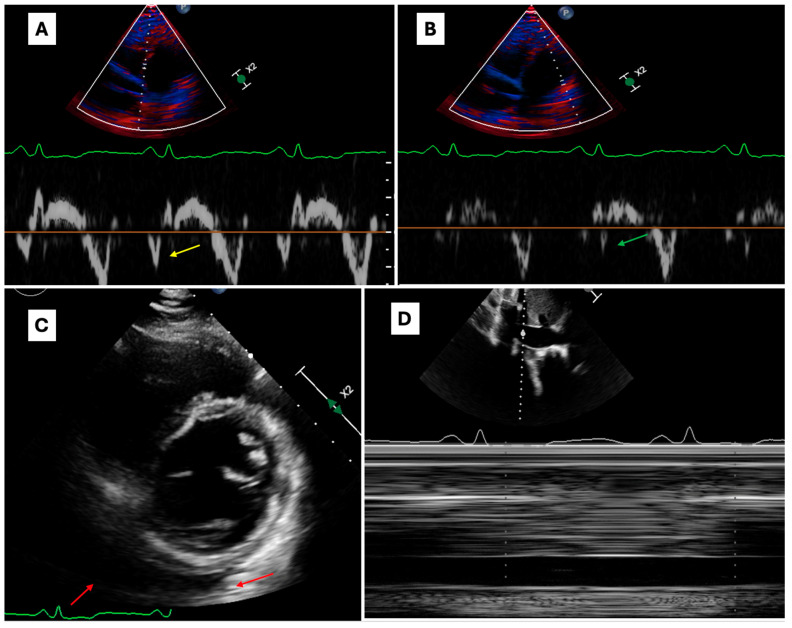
Echocardiographic findings of a patient with constrictive pericarditis. Echocardiographic findings of a patient with long-standing worsening right-sided heart failure. Panels (A,B) show an “annulus reversus” pattern on tissue Doppler imaging (TDI) evaluation (medial e’ velocity, yellow arrow; > lateral e’ velocity, green arrow; on same velocity reference scale). Panel (C) shows moderate circumferential pericardial effusion, more represented at the left ventricular posterior wall (red arrows). Panel (D) shows dilated and hypo-collapsible inferior vena cava.
